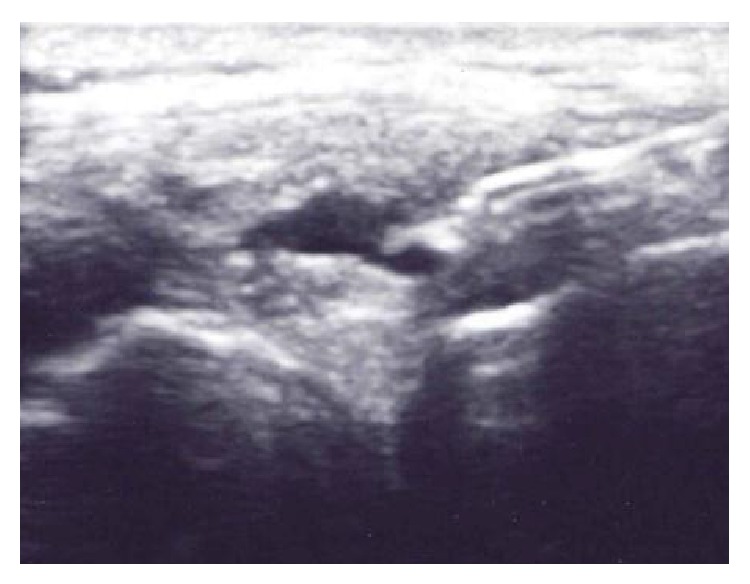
Ultrasound image in the coronal plane demonstrating aspiration of the meniscal cyst (arrow).
